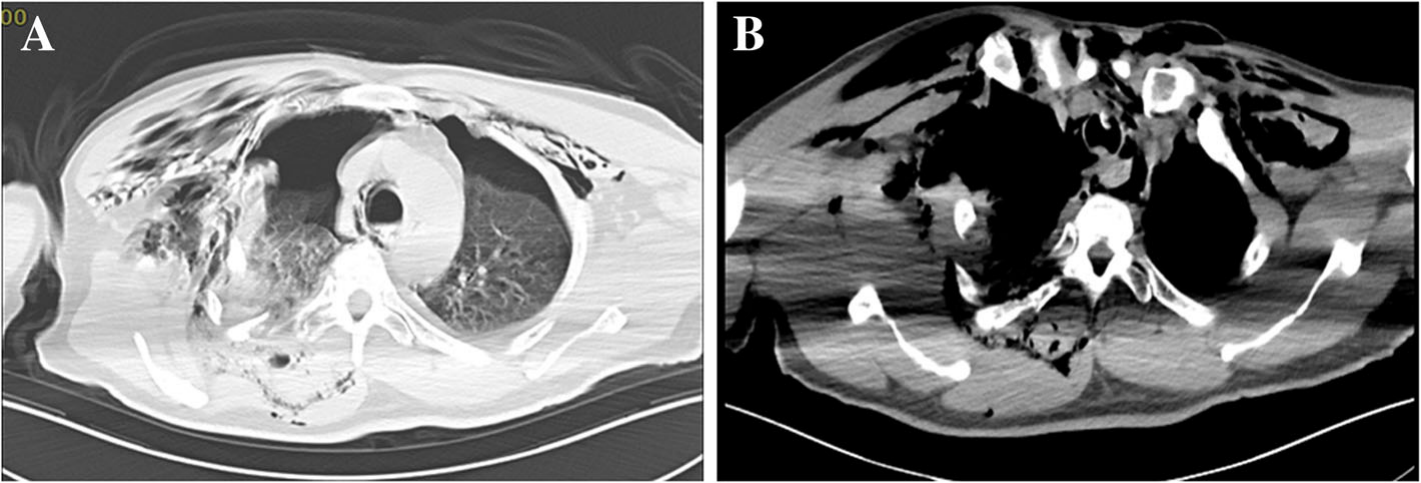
Thoracic CT scan showing massive subcutaneous emphysema, pneumomediastinum, multiple rib fractures, bilateral hemopneumothorax and compressive pneumothorax on pulmonary (a) and mediastinal (b) window
